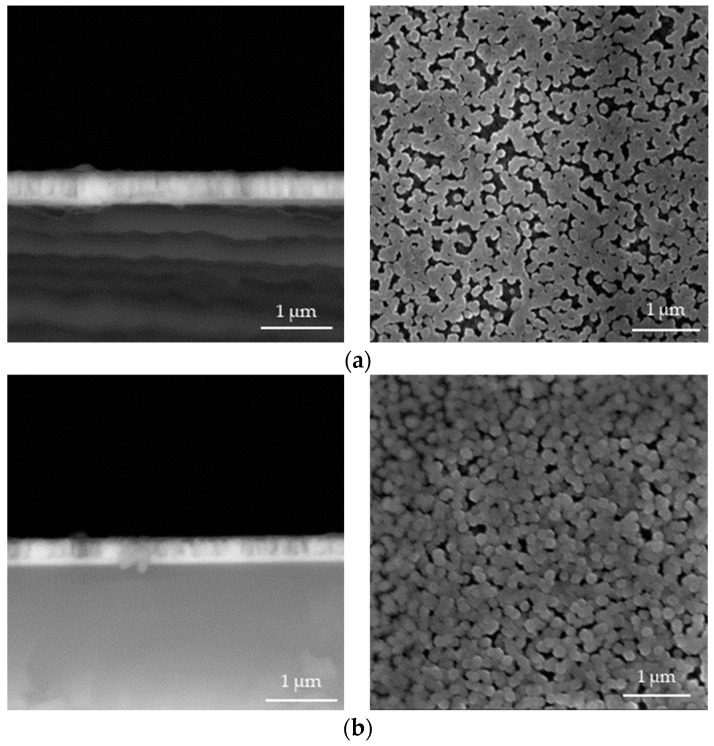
SEM images of nanorods obtained with 3 min of ultrasound and dried for 1.5 h (a) and 2.0 h (b) (left: cross-section image; right: surface image).
